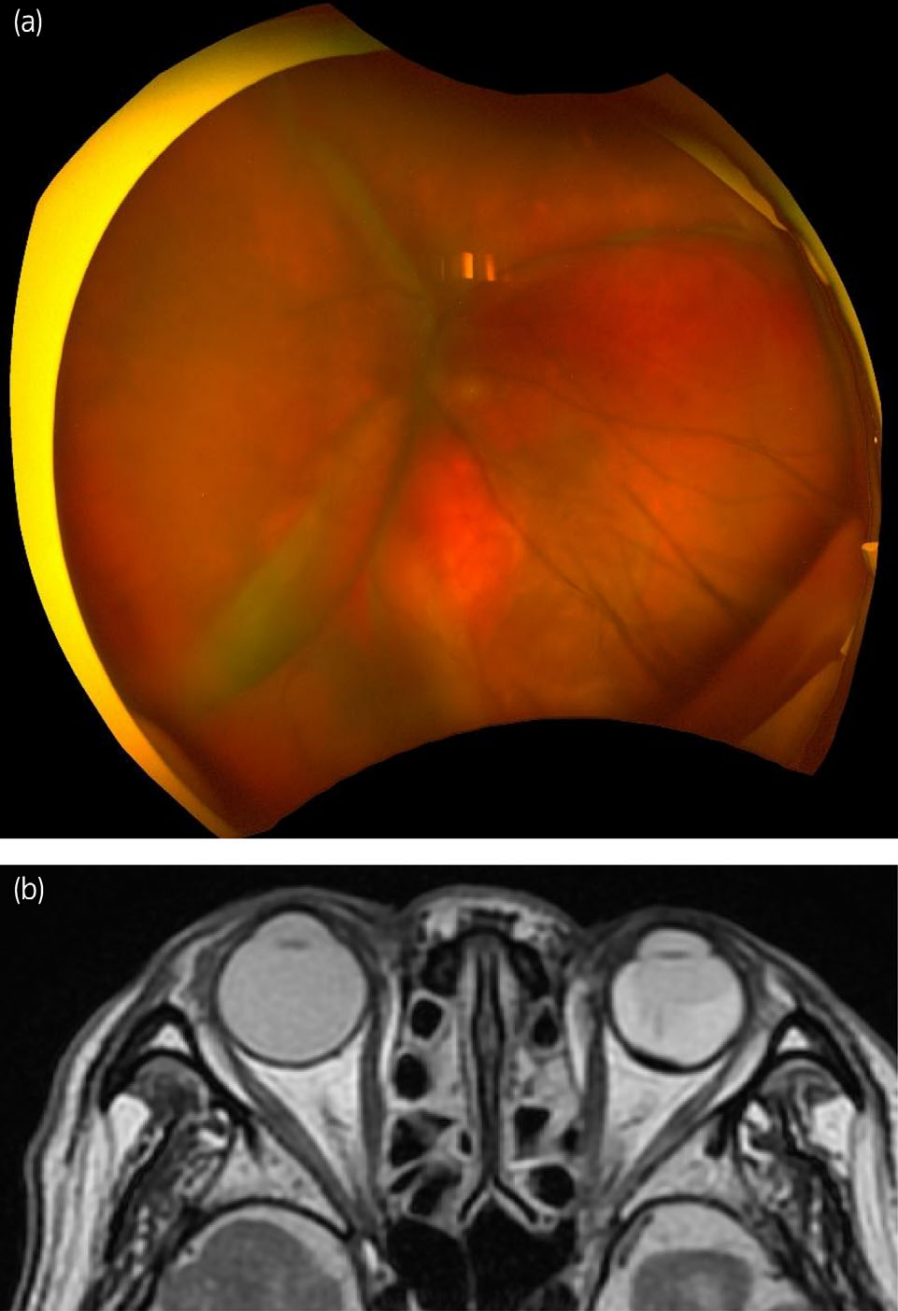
(a) Fundus examination after treatment found no tumor but the retinal detachment persisted. (b) T2‐weighted axial magnetic resonance imaging (MRI) showing that the choroidal metastasis treated with radiotherapy at 30 Gy gradually disappeared over 15 months.
